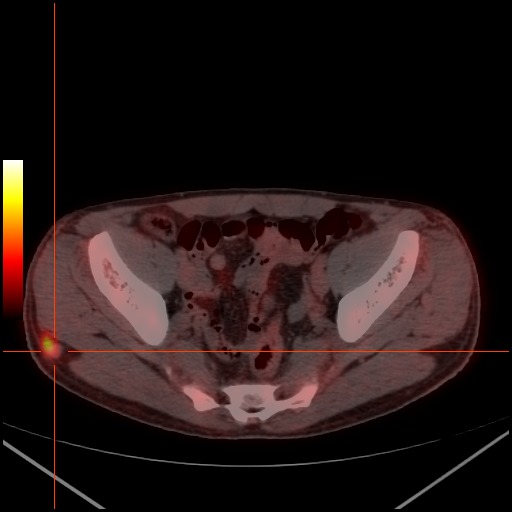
Foyer hyperméabolique (SUVmax bw: 4.3) dans la région fessière droite
